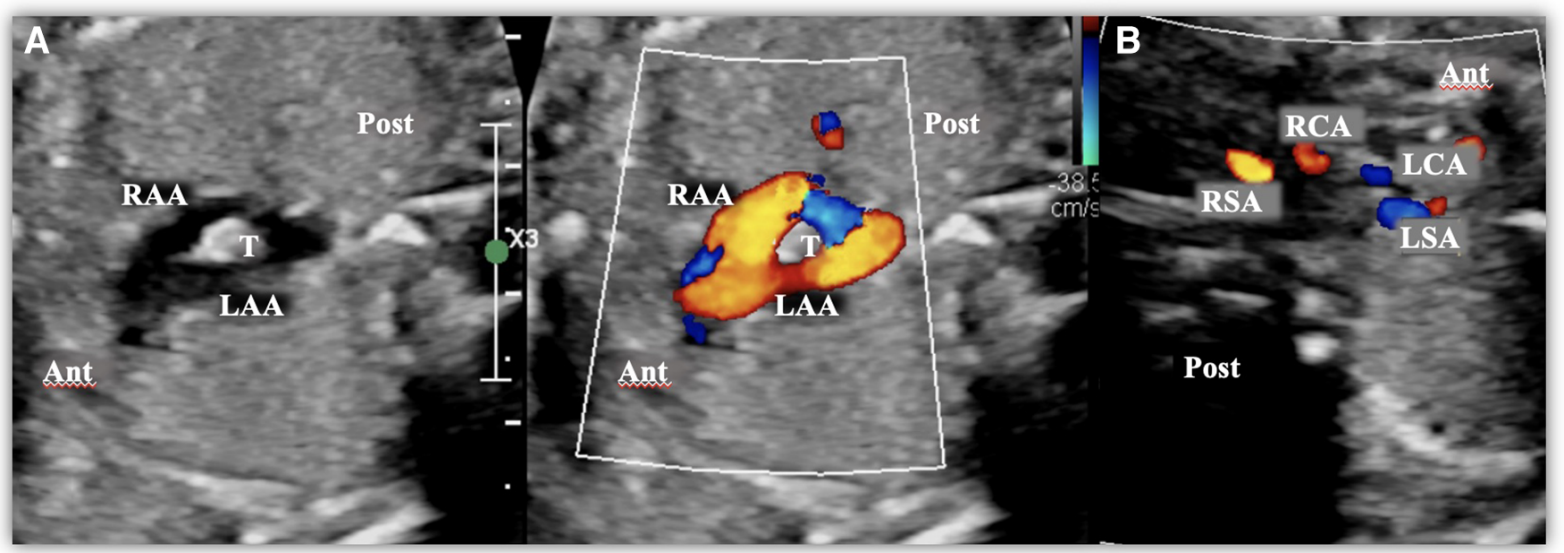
Images taken from a fetal transthoracic echocardiogram. (A) A cross-sectional view at the level of the aortic arch, with a color Doppler, where it is possible to observe the complete vascular ring surrounding the trachea, consisting of a double aortic arch. (B) A cross-sectional view at the level of the epiaortic vessels, with a color Doppler, showing the four epiaortic vessels originating separately (two on the right and two on the left). Ant, anterior; LAA, left aortic arch; LCA, Left carotid artery; LSA, left subclavian artery; Post, posterior; RCA, right carotid artery; RSA, right subclavian artery; T, trachea.
